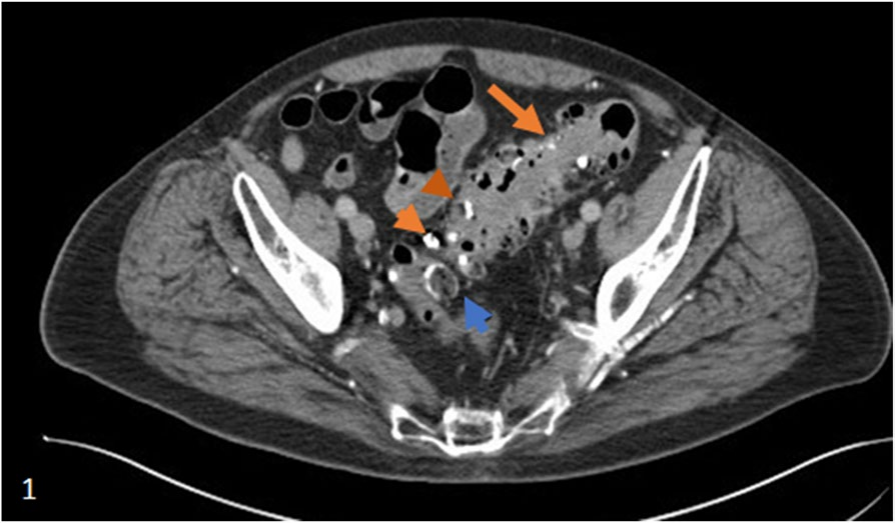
Axial CT image of the pelvis showing multiple sigmoid colonic diverticula (orange arrow). Many of these diverticula demonstrate markedly hyperattenuating material filling them (orange arrowheads) which was proven to be barium sulfate after resection of the colon. There is a sigmoid anastomotic donut in trans-axial section (blue arrow) with hyperattenuating material within it as well. Evidence of classic gross barium material along the peritoneal surfaces was not seen in this case
